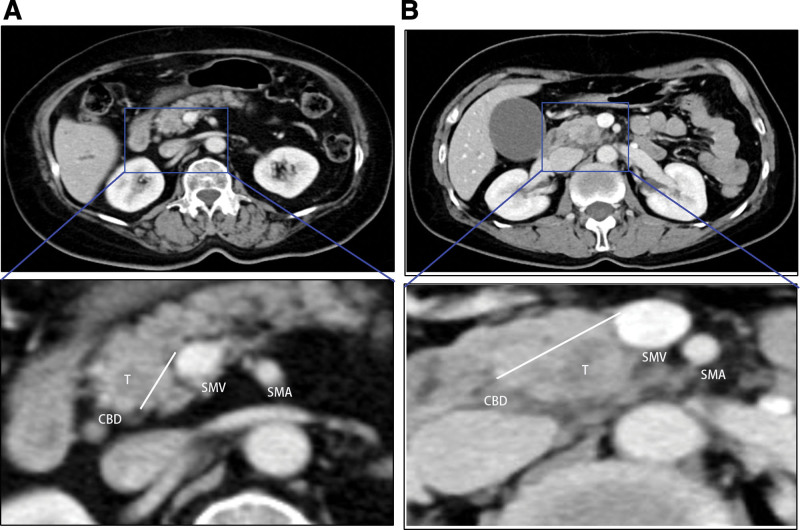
CT scan distinguishes the tumor deriving from dorsal or ventral pancreases. (A) Following the above standard, The CT scan image of pancreatic head cancer arising from dorsal pancreas. (B) The CT scan image of pancreatic head cancer arising from ventral pancreas. CBD = common bile duct, CT = computerized tomography, SMV = superior mesenteric vein, SMV = superior mesenteric artery, T = tumor.
